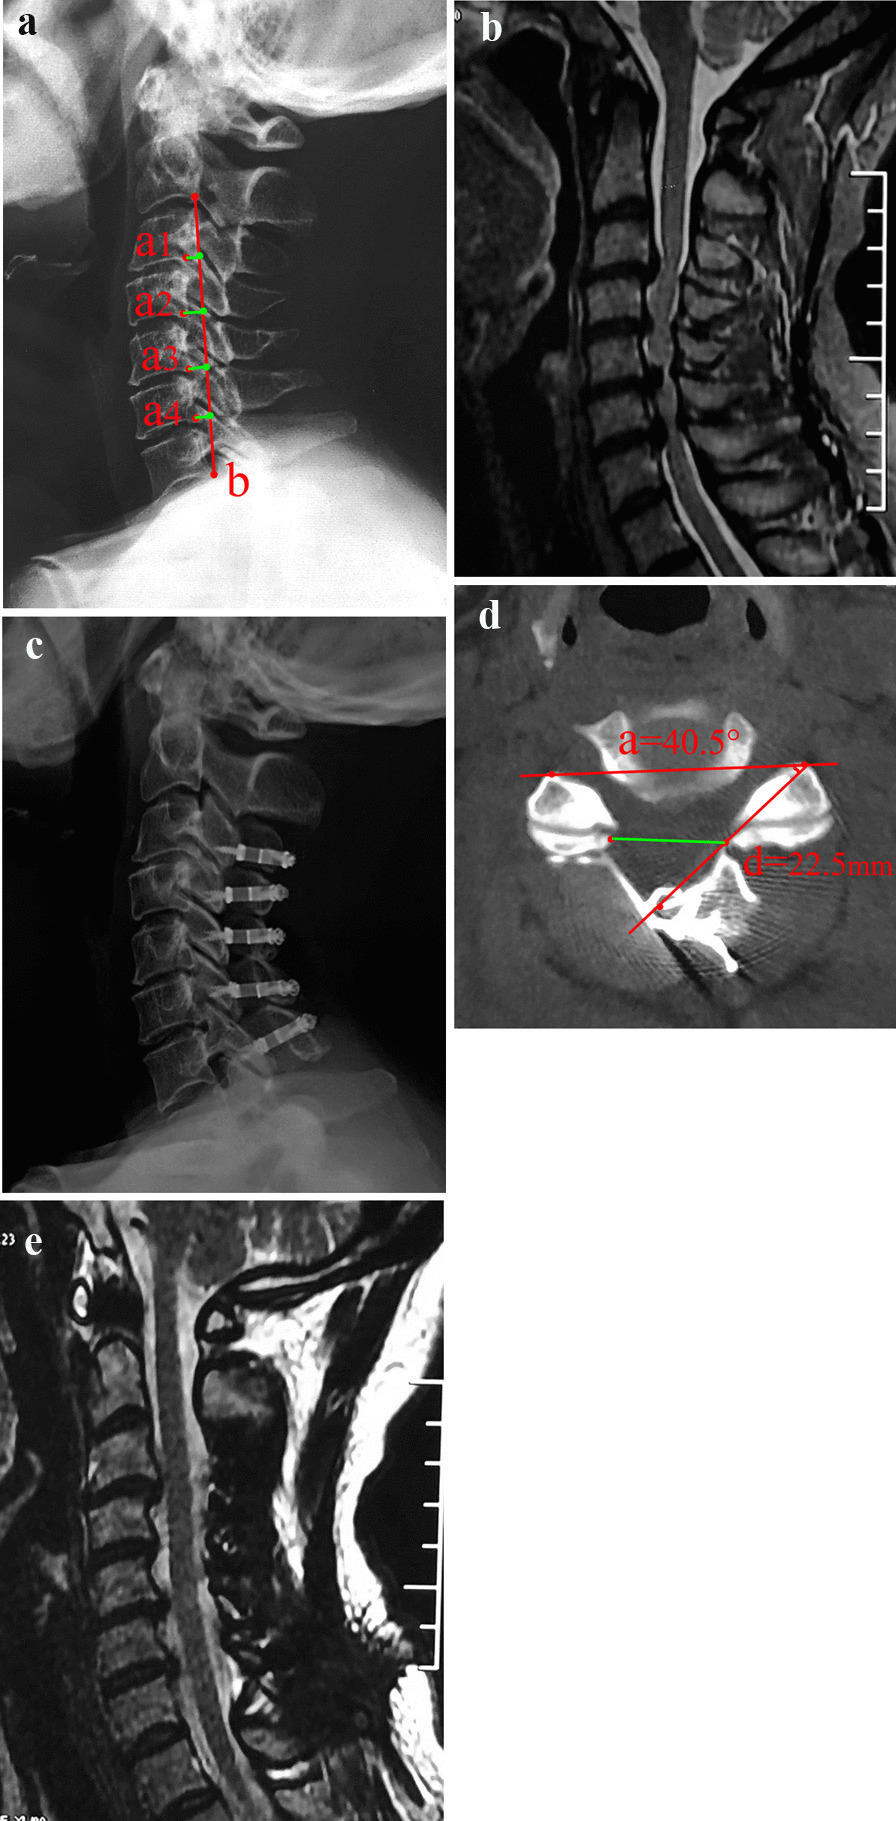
A 63-year-old male patient had typical symptoms of cervical spondylotic myelopathy for 16 months. a Preoperative X-ray showing the same cervical degeneration. b MRI showing serious spinal stenosis at the C3-7 segments. c Postoperative X-ray showing C_3-7_ open-door laminoplasty with titanium plate fixation. d Postoperative CT showing the titanium plate fixed on the lateral mass, the opening angle was 40.5° and the opening width d was 22.5 mm. e Postoperative MRI showing adequate decompression, and the spinal cord drift distance was 2.7 mm
